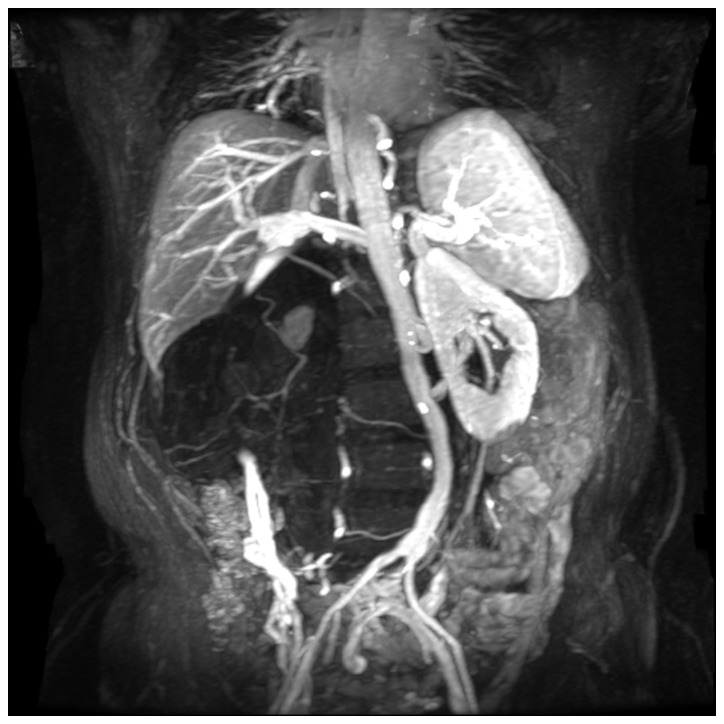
Magnetic resonance imaging (MRI) revealing the location and morphology of the tumor and the adjacent structures. The abdominal aorta was crushed to left and the inferior vena cava was narrowed. The ascending lumbar, azygos and hemiazygos veins were distended and twisted markedly.
